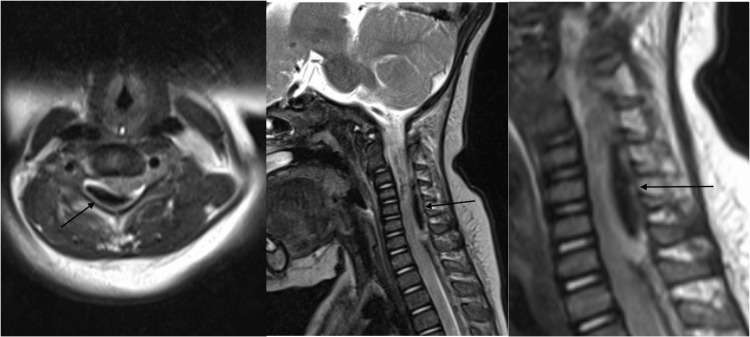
MRI T2-weighted image of axial and sagittal views showing a posterior hematoma from the C2 to T8 level predominantly at the C4 to T1 level causing canal stenosis. MRI: magnetic resonance imaging
